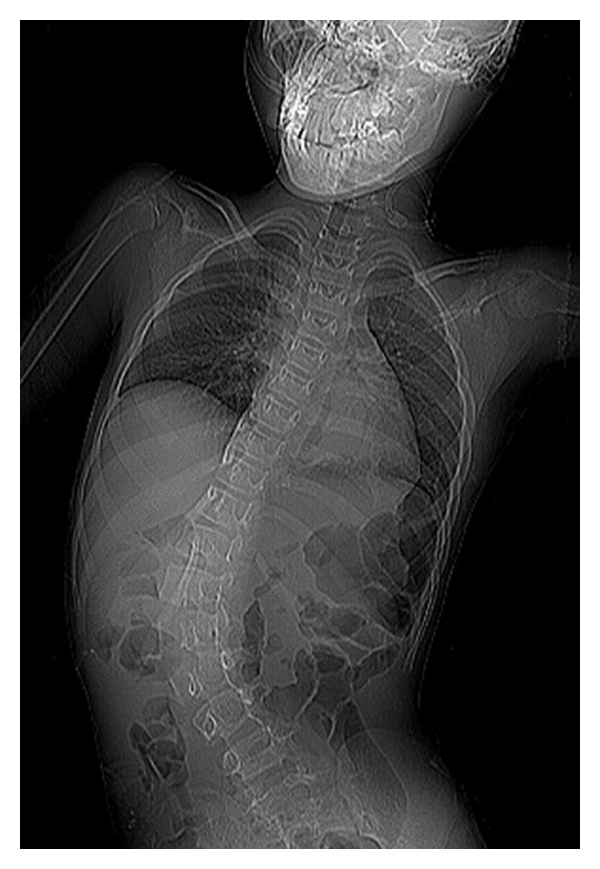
Chest X-ray image shows severe scoliosis.
